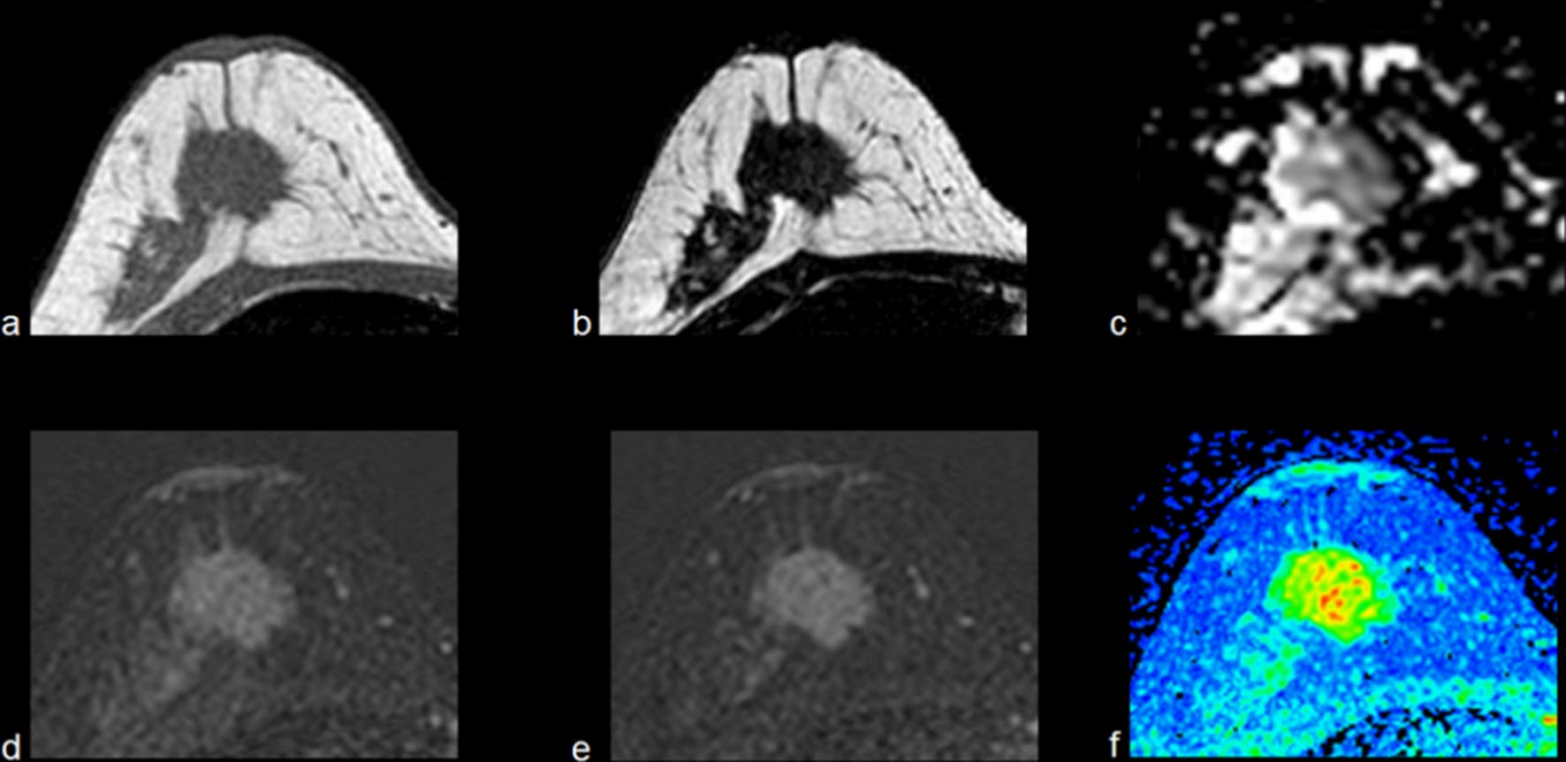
Example of MRI finding of breast lesion classified as BIRADS 4A by echography. A 44-years-old patient with a solid mass with irregular contours at the right breast presents superior external spiculations. It appears hypointense on T1W (a) and T2W (b), and ADC (c) value of 0.8 × 10^−3^mm^2^/s. At initial (d) and delayed (e) postcontrast subtraction images, it shows a homogeneous gradual enhancement (f) with a wash-out pattern. Thus, it was classified as BIRADS 5. The pathological analysis confirmed it as Invasive Ductal Carcinoma
